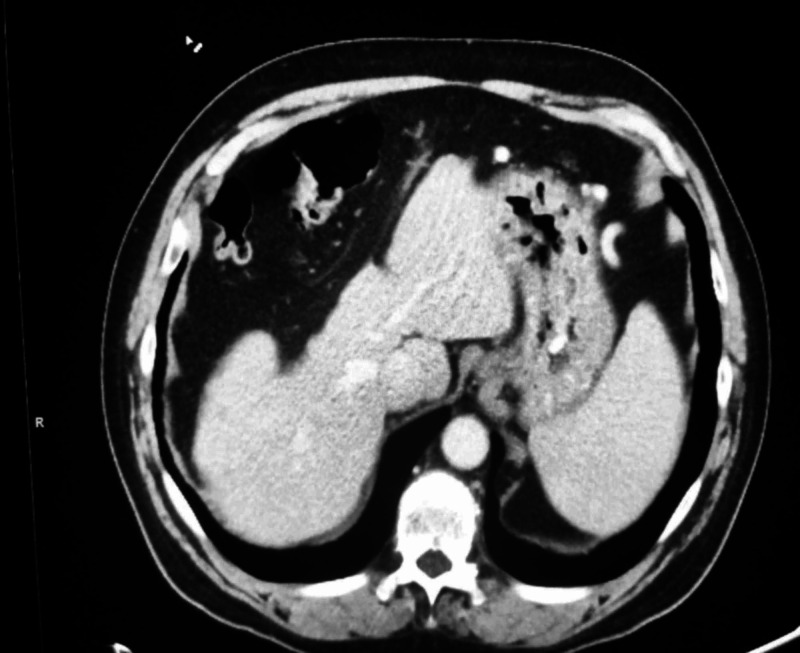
Axial view of a CT scan of the abdomen and pelvis with contrast demonstrates complete resolution of the previously observed Streptococcus mitis abscesses.
